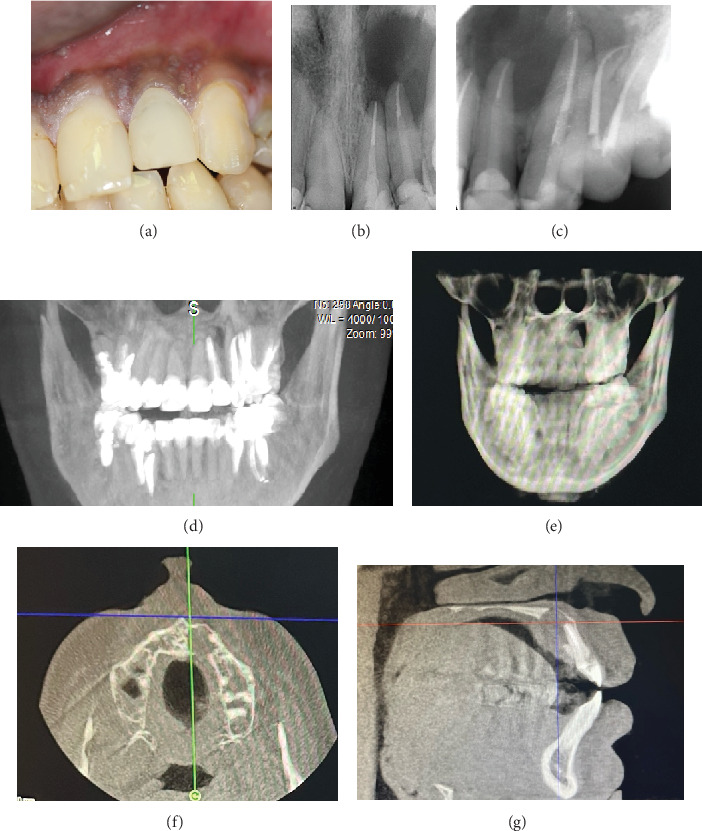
(a) Clinical preoperative photograph. (b, c) Preoperative periapical radiographs (straight and shift views) showing the periradicular lesion involving both Teeth #21 and #22 previously endodontically treated. (d, e) Panoramic image of CBCT of anterior area. (f, g) CBCT slices showing the periradicular through-and-through radiolucency related to treated #21 and #22.
